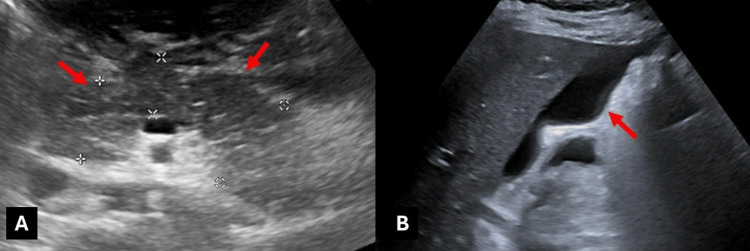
Abdominal ultrasound images showing increased thickness and decreased echogenicity of the pancreatic parenchyma (arrows in A), with free fluid in the abdomen predominantly around the liver (arrow in B).
